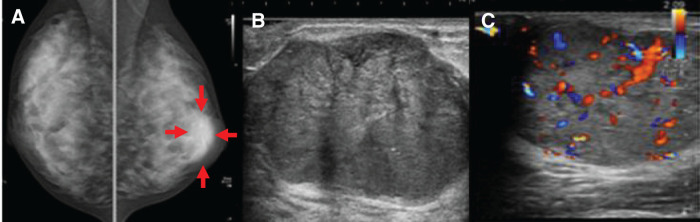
(A) Mammography: a mass just below the nipple in the left breast (arrows). (B) Ultrasonograpy: a lobular hypoechoic mass, 53 mm in size with multiple slit-like structures inside the mass. (C) Ultrasonography: blood flow inside the mass was rich.
